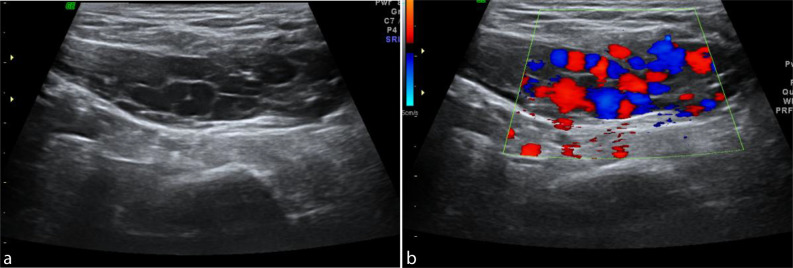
(a) Grey-scale sonogram along the longitudinal plane showing tubular tortuous anechoic channels in the right inguinal region. (b) Colour Doppler image showing flow signals in the vascular channels.
